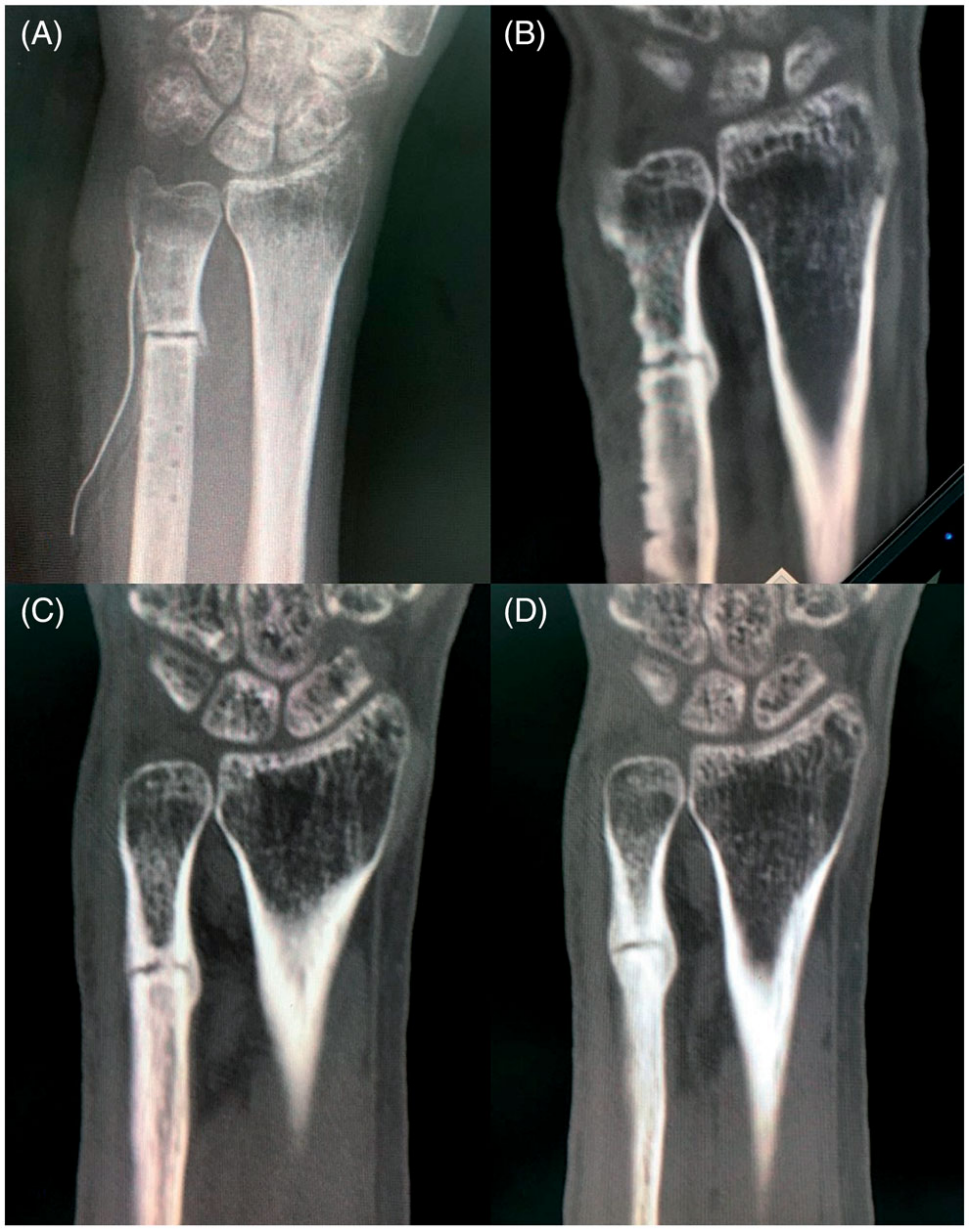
(A) Postoperative X-ray (immediately after implant removal and debridement). (B) Postoperative computed tomography (CT, 1 month after implant removal and debridement). There was poor callus formation and a bony absorption at the osteotomy site. (C) Postoperative CT (4 months after implant removal and debridement and 3 months after the start of teriparatide therapy and low-intensity pulsed ultrasound [LIPUS]). (D) Postoperative CT (5 months after implant removal and debridement and 4 months after the start of teriparatide therapy and LIPUS). Bony union at the osteotomy site was observed.
